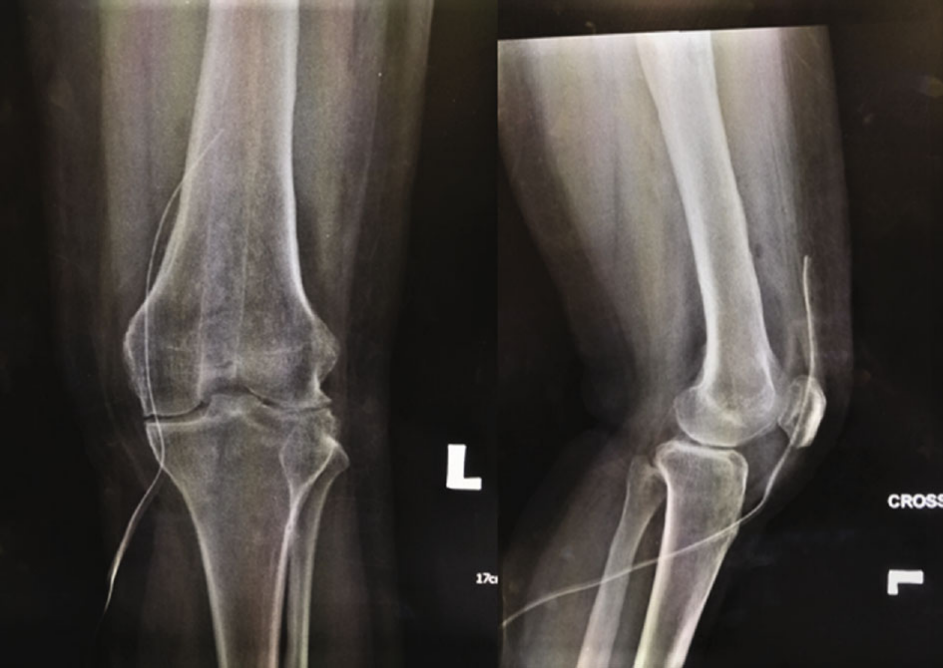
X-rays of left knee post K-nail removal.
